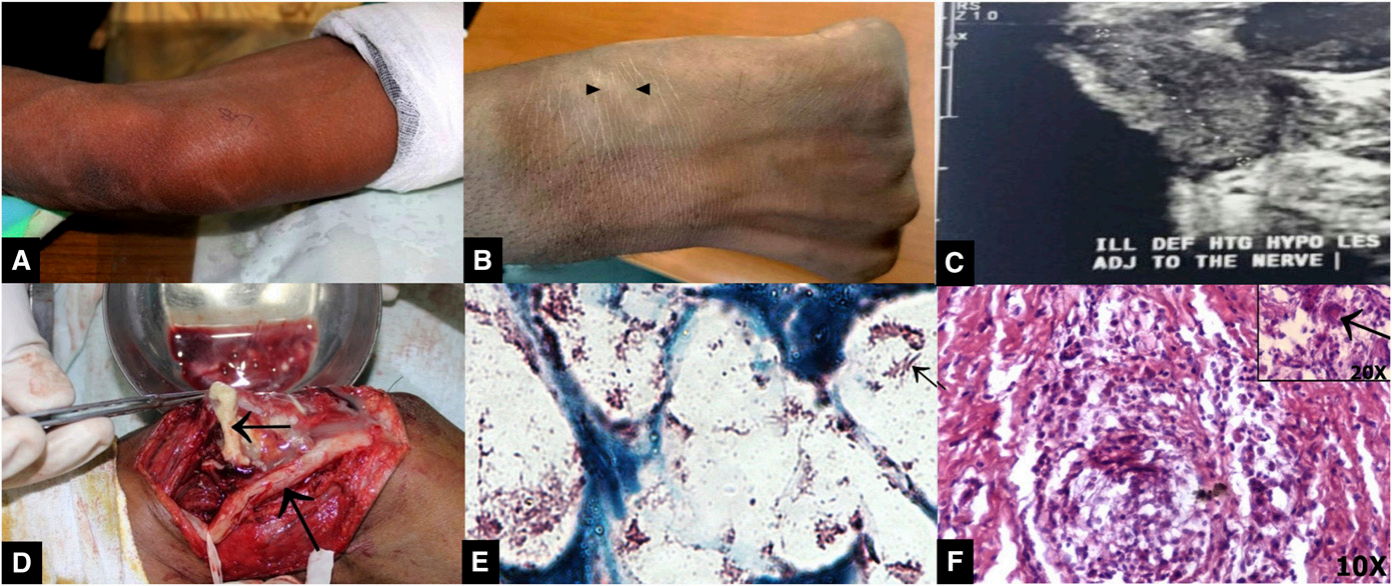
Image showing swelling and signs of inflammation at the ulnar aspect of the lower arm region (A), enlargement of the left superficial radial nerve (B), ultrasound depiction of subcutaneous and ulnar nerve abscesses (C), operative image showing the thickened ulnar nerve and pus drained from swelling (D), acid-fast bacilli (E), and Langhans giant cells (F). This figure appears in color at www.ajtmh.org.
